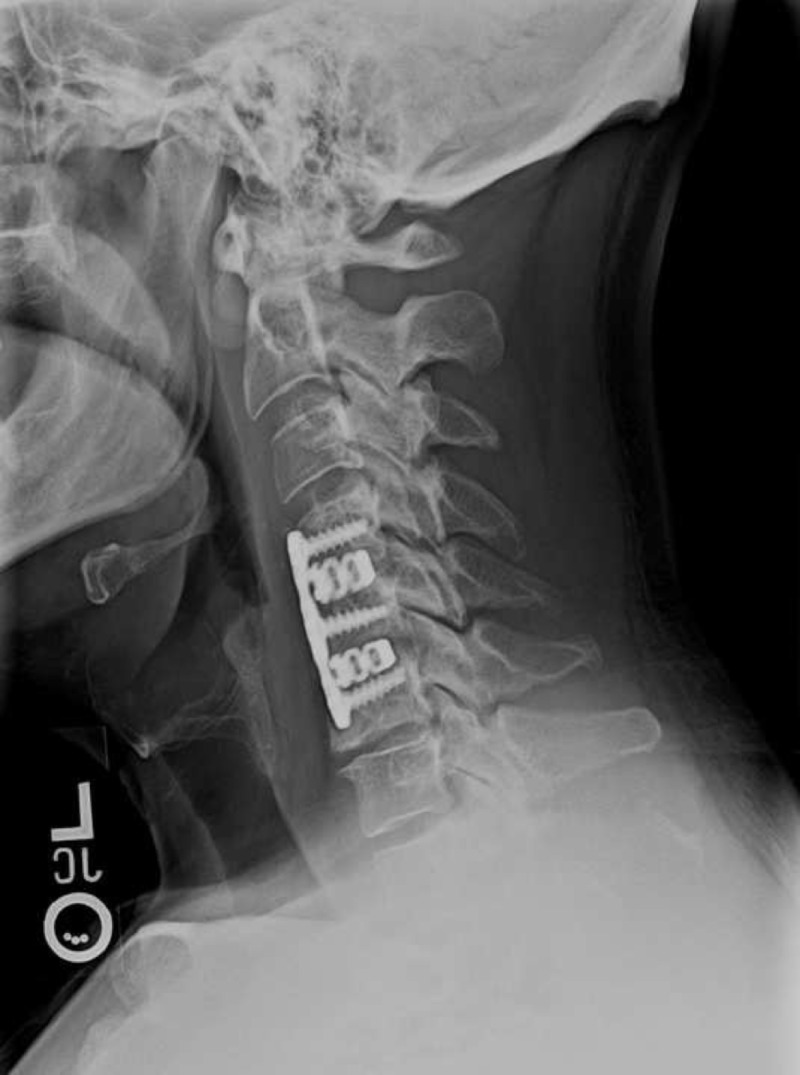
Lateral X-ray of cervical spine at nine months
